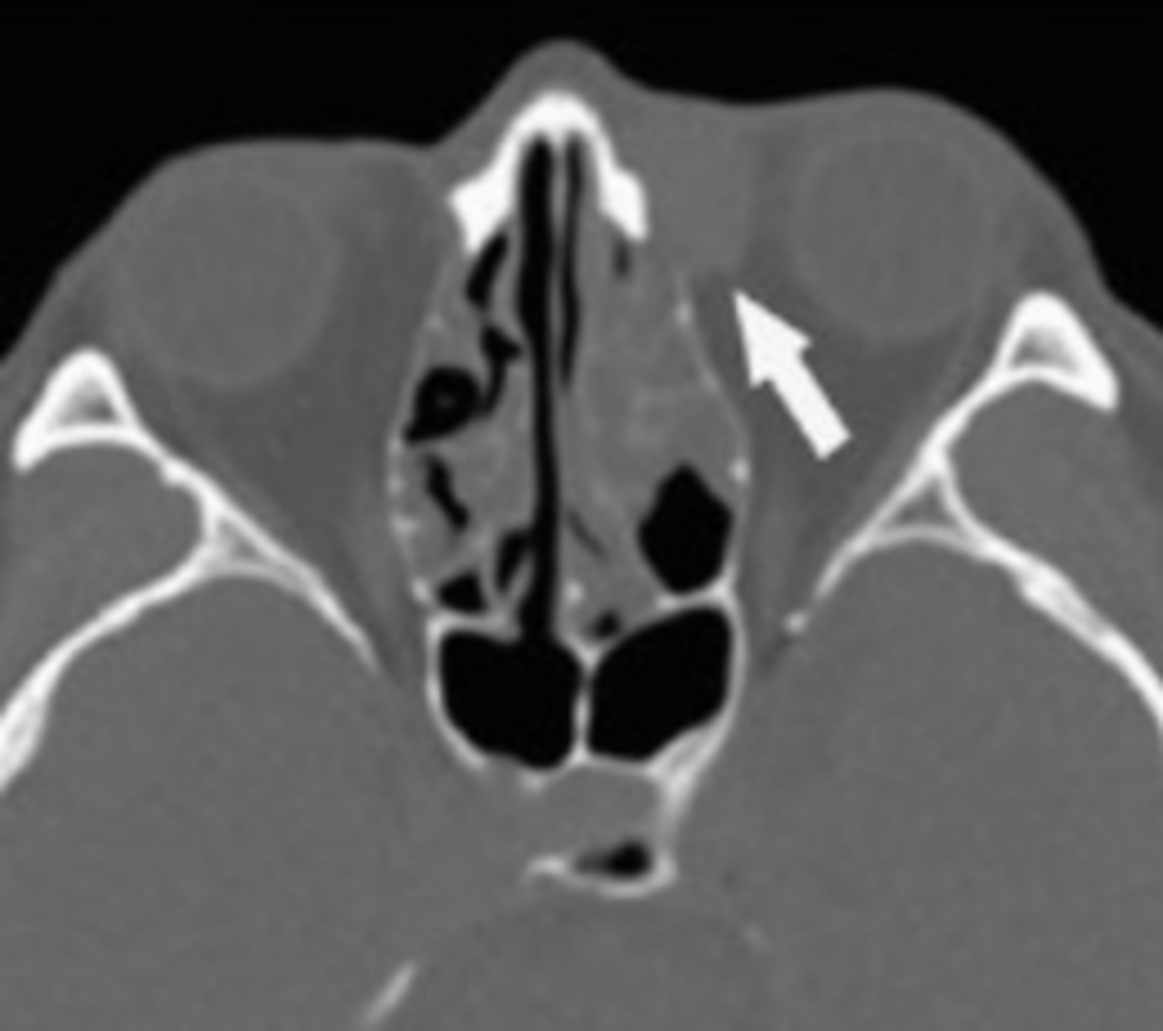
Computed tomography demonstrating homogeneous soft tissue density in the medial surface of the globe indicated by the tip of the arrow.
